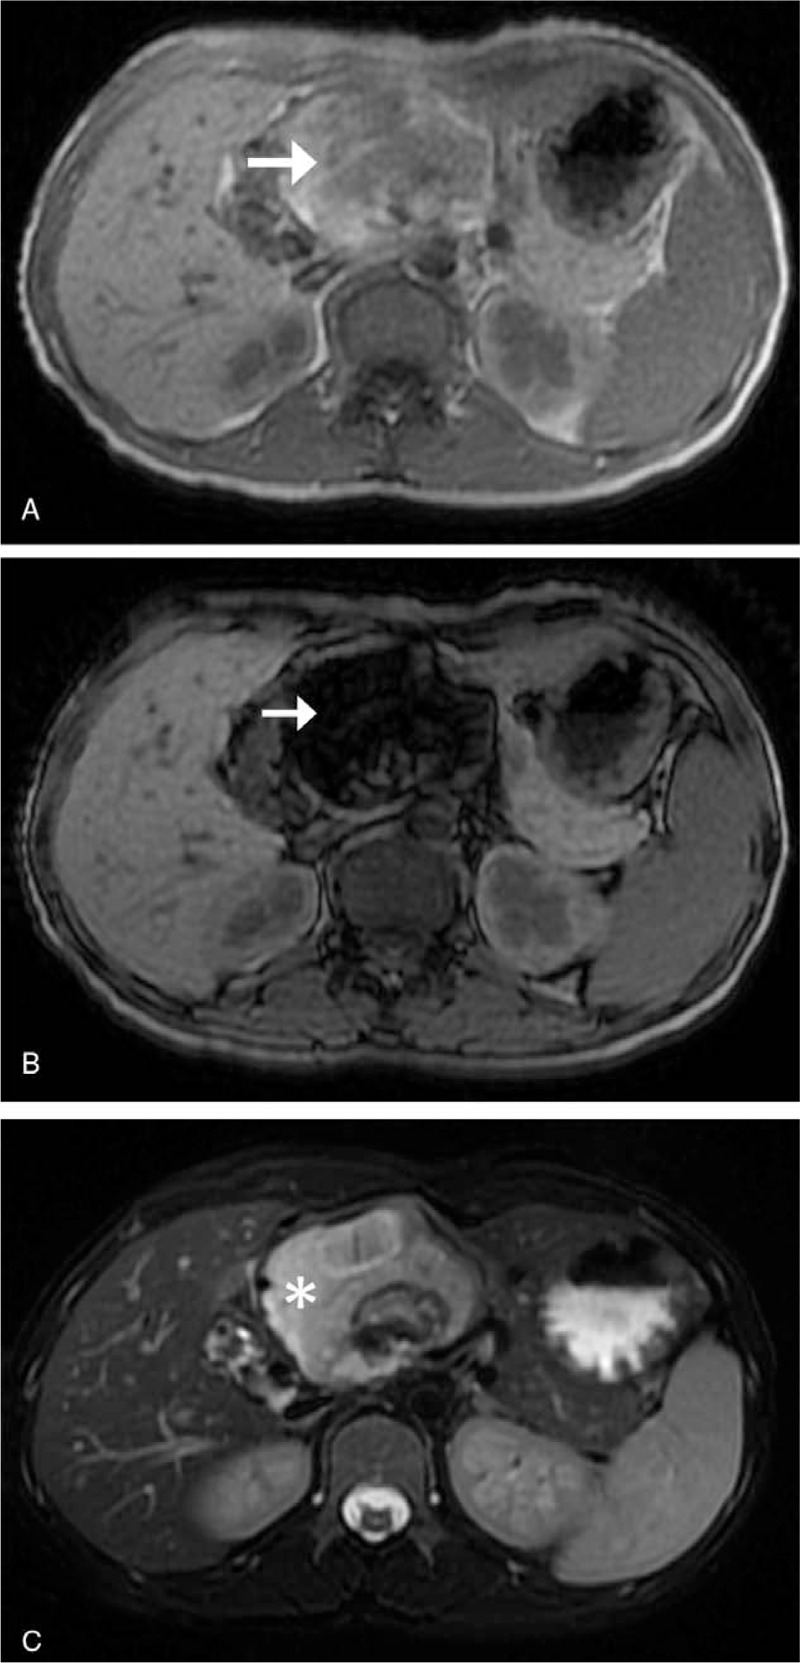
Magnetic resonance imaging (MRI) (A) In-phase sequence showing heterogeneous high signal intensity mass (arrow) in the head of the pancreas. (B) Out-of-phase sequence showing a drop out (low) signal (arrow). (C) T2-fs showing a heterogeneous high signal.
